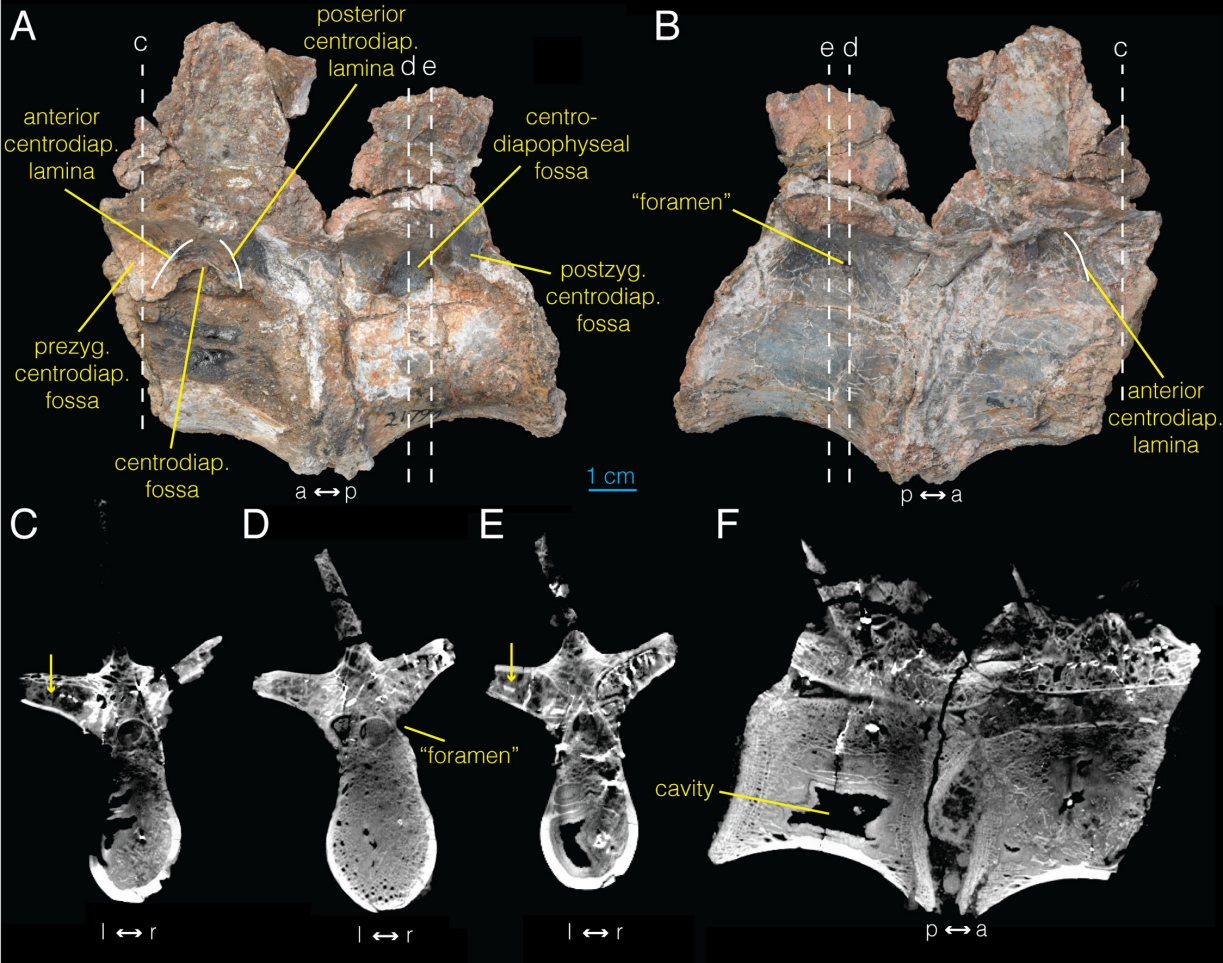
Sacral vertebrae of *Archaeornithomimus* (AMNH FARB 21790) and associated CT images. A, left lateral view; B, right lateral view; C–E, select transverse sections; F, midsagittal section. Dashed lines and associated letters indicate location and letter designation of CT image slices.
